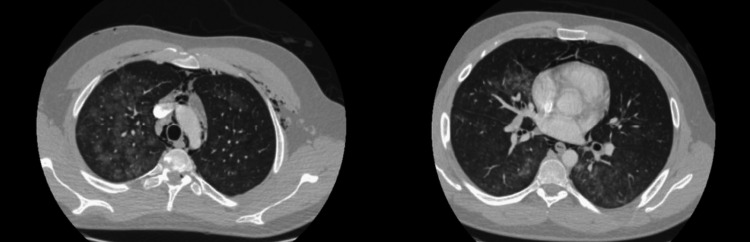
Diffuse ground glass opacities on a CT of the chest of a chronic vape user.
